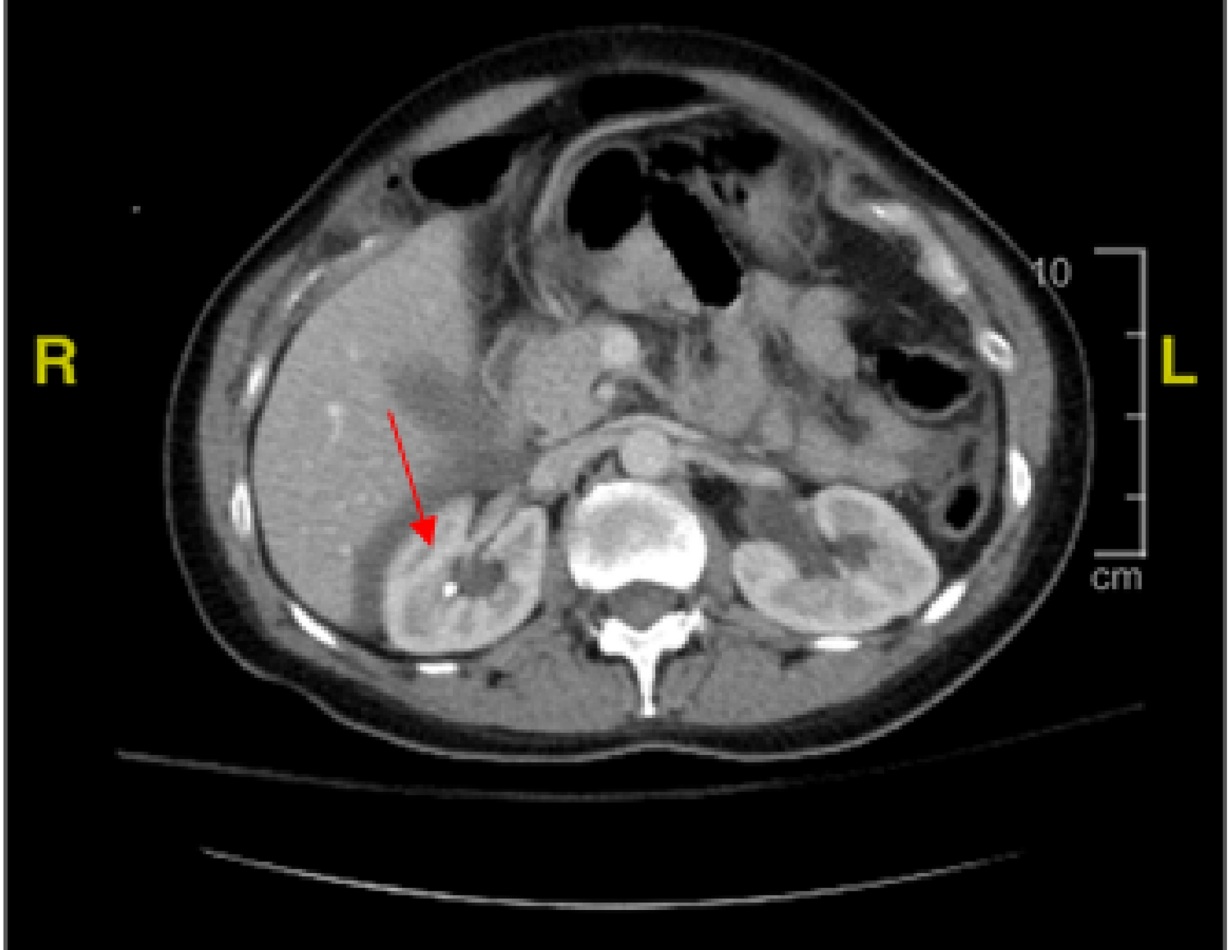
CT scan showing nephrolithiasis in the right kidney
